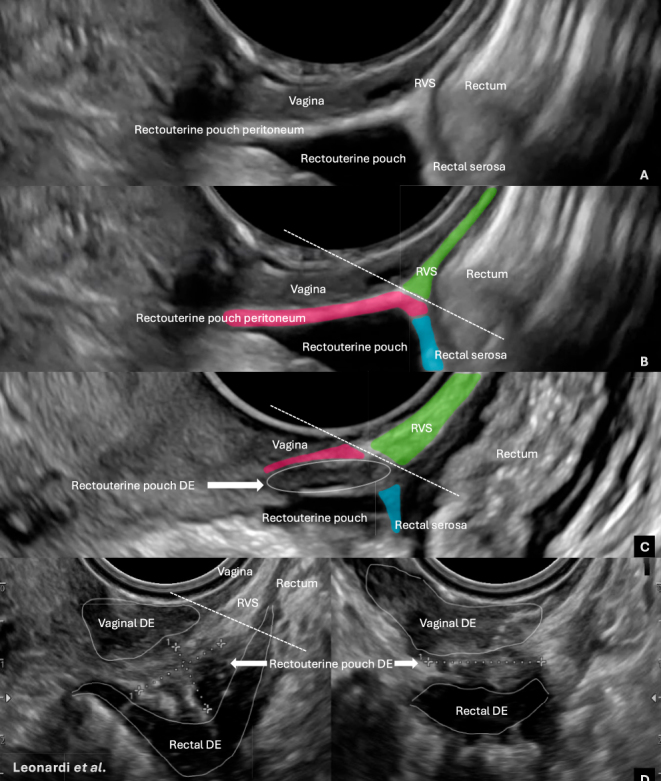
Sonographic depiction of the posterior compartment anatomical structures, normal (A and B) and with DE (C and D). (A) Normal anatomy without colour overlays depicting the vagina (hypoechoic band closest to the ultrasound probe), rectouterine pouch peritoneum overlying the vagina (hyperechoic thin tissue band), rectovaginal septum (hyperechoic band, contiguous with rectouterine pouch peritoneum, between the vagina and retroperitoneal rectum), rectum (mixed echogenic features with various layers), and overlying intraperitoneal hyperechoic rectal serosa. (B) Matching normal anatomy with colour overlays. (C) Non-obliterated rectouterine pouch (i.e. no adhesions), with DE within the rectouterine pouch peritoneum, above and at the peritoneal reflection, but without infiltration into the RVS. (D) Severe posterior compartment DE with three distinct nodules within the vagina, rectouterine pouch peritoneum, again above and at the peritoneal reflection, and intraperitoneal rectum. The adhesions, i.e. obliteration between the rectum and the vagina via the rectouterine pouch peritoneum, give the impression that the central DE lesion is within the retroperitoneum. Understanding the division between the RVS and the peritoneal reflection (even when obliteration exists) permits the understanding that the RVS is essentially preserved in severe posterior compartment endometriosis cases.
